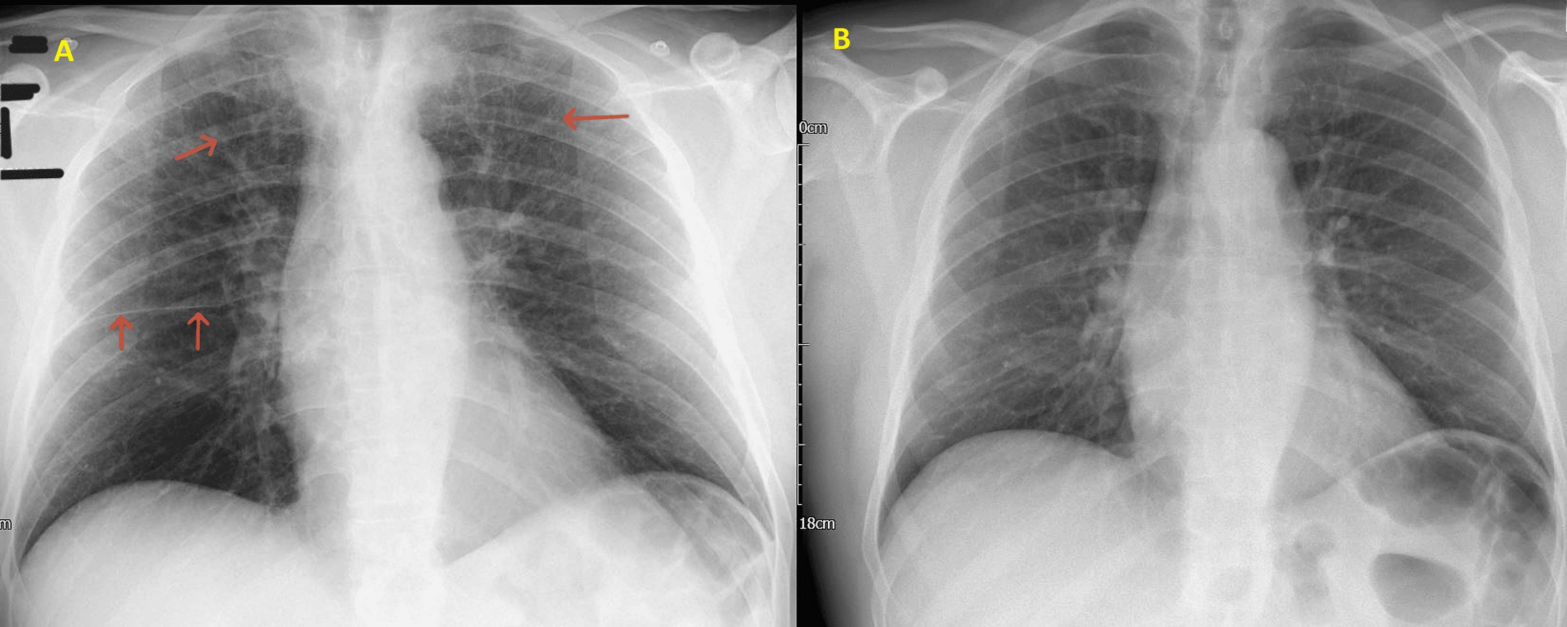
Post-angiogram CXR (A) Post-angiogram CXR showing accentuated interstitial markings in both upper zones (orange arrows at the apices) and fluid within the right horizontal fissure (Kerley B lines, red arrows). (B) Comparison with the patient’s normal CXR two months earlier, showing no abnormal findings. CXR, chest X-ray
